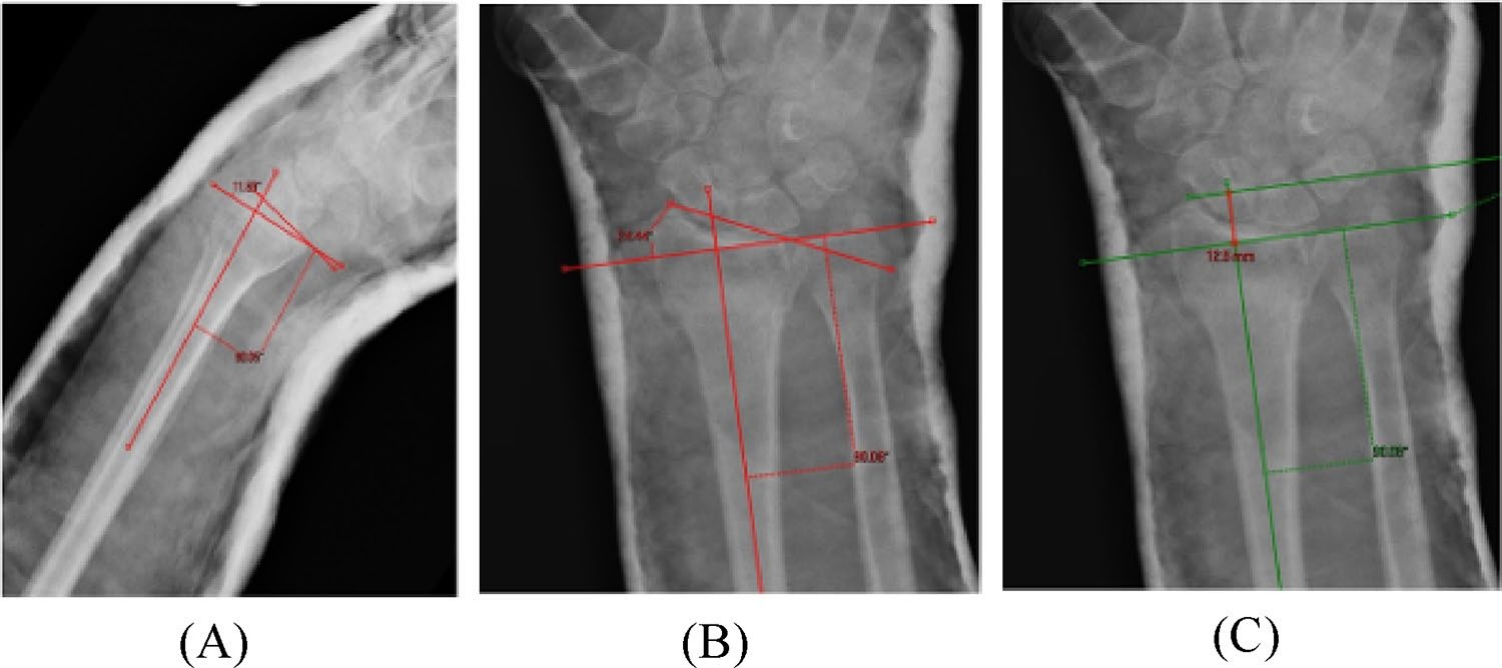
A Measurement of Dorsal Angulation on Lateral Radiograph; B Measurement of Wrist Inclination on PA Radiograph; C Measurement of Wrist Length on PA Radiograph;
